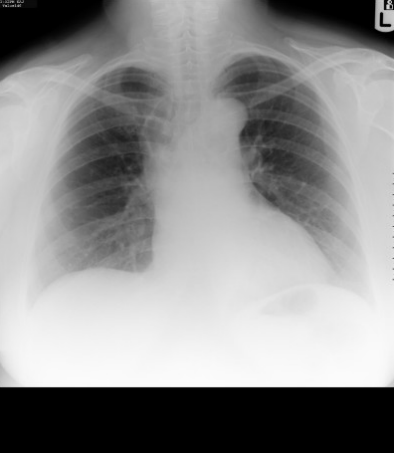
Chest radiograph showing the air-filled mediastinal mass.
